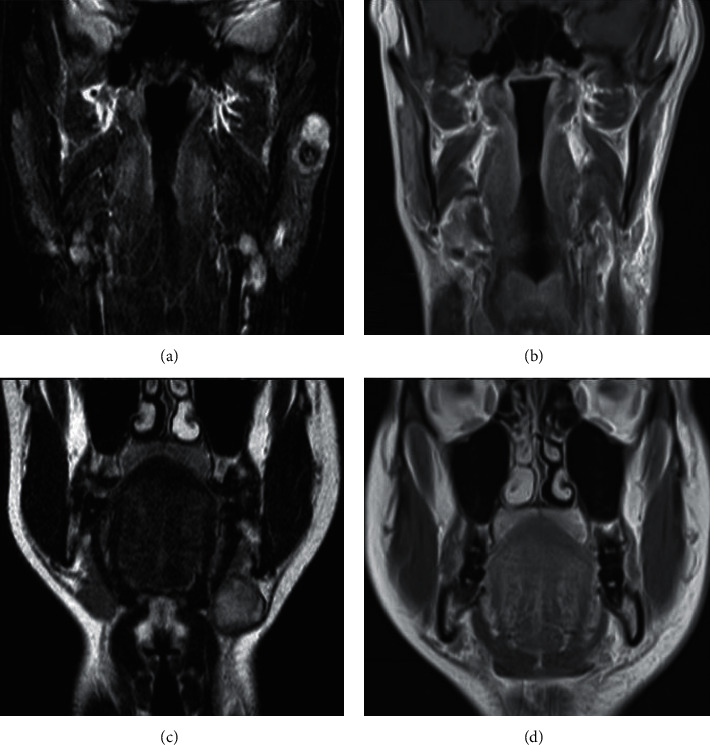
(a, c) Presurgery contrast-enhanced MRI of the head and neck; (b, d) 3-month follow-up MRI.
